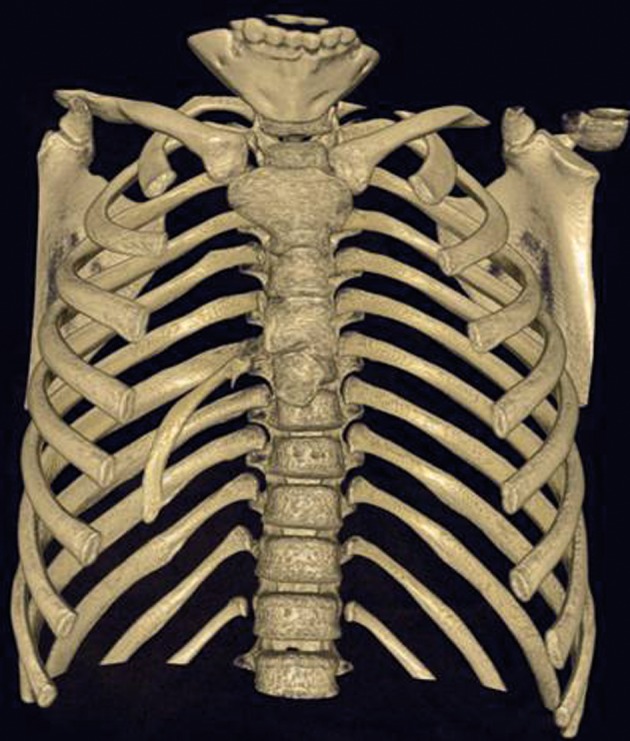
Spiral CT, anterior appearance. A right intrathoracic rib which is articulated on the anterior aspect of the T7 vertebral body and protrudes into the thorax cavity causing collapse of the lower lung parenchyma
